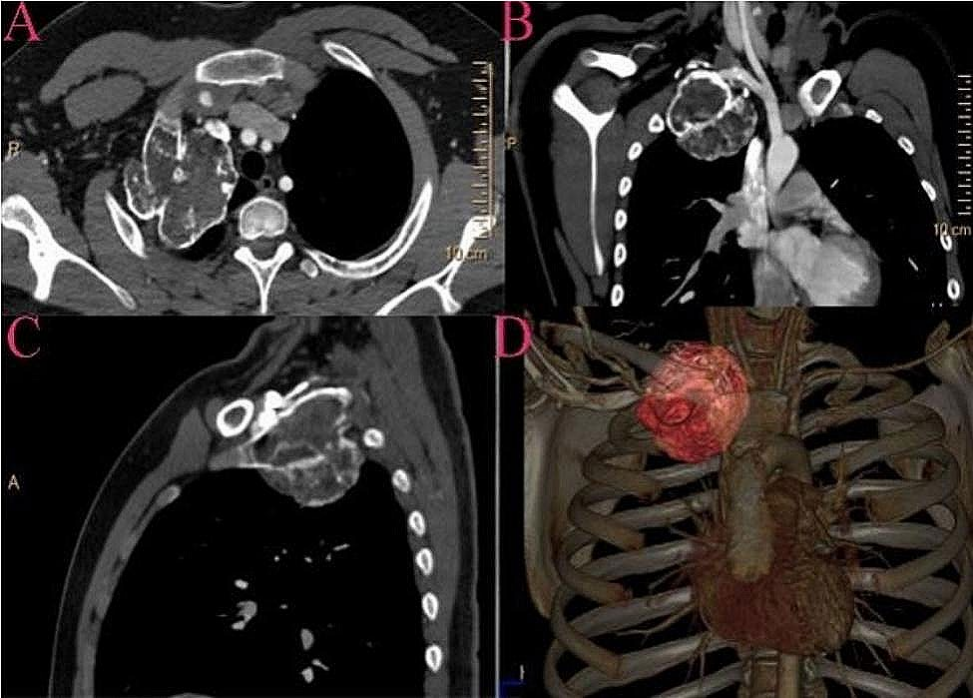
Chest computed tomography shows a bulging calcified mass arising from the right first rib. (A) Axial view. (B) Coronal view. (C) Sagittal view. (D) Chest 3D-CT images
